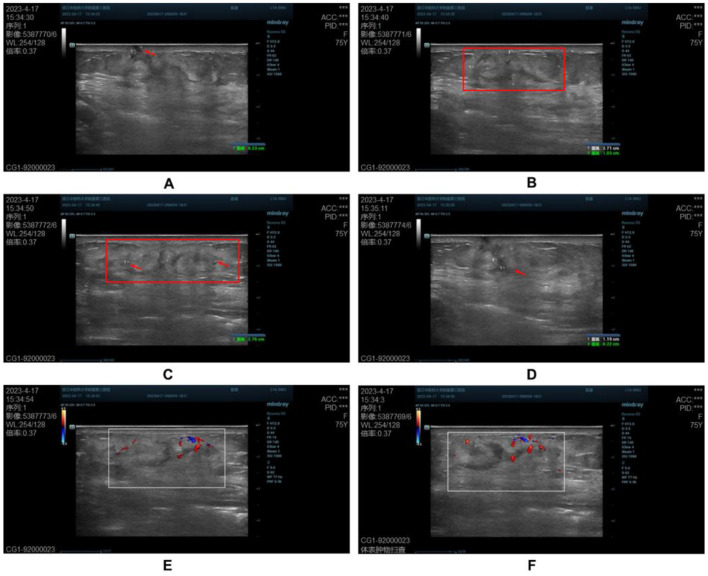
Ultrasonography on the incisional infection site. (A) means the tube to the skin; (B–D) mean the size of the incisional infection site; (E and F) mean the blood flow in the incisional infection site.
